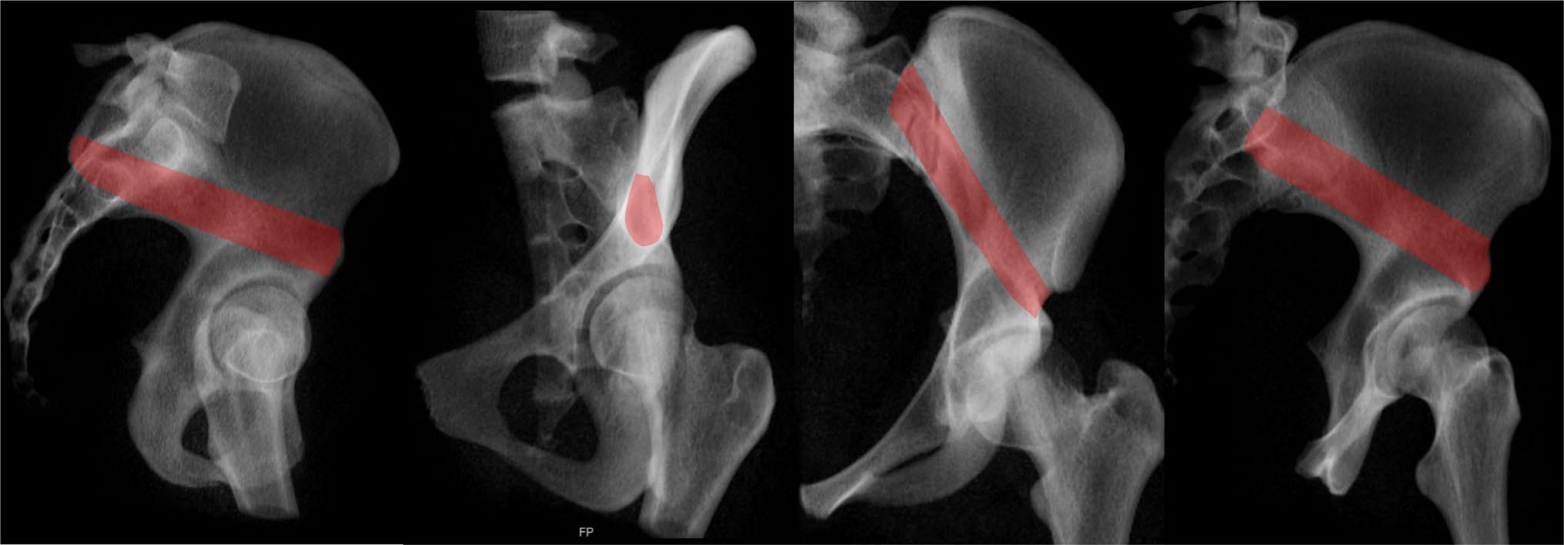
Radiographic visualization of the supraacetabular corridor (SAC) using the true lateral view, COOO, pelvic Inlet and CIOO views (from left to right)
